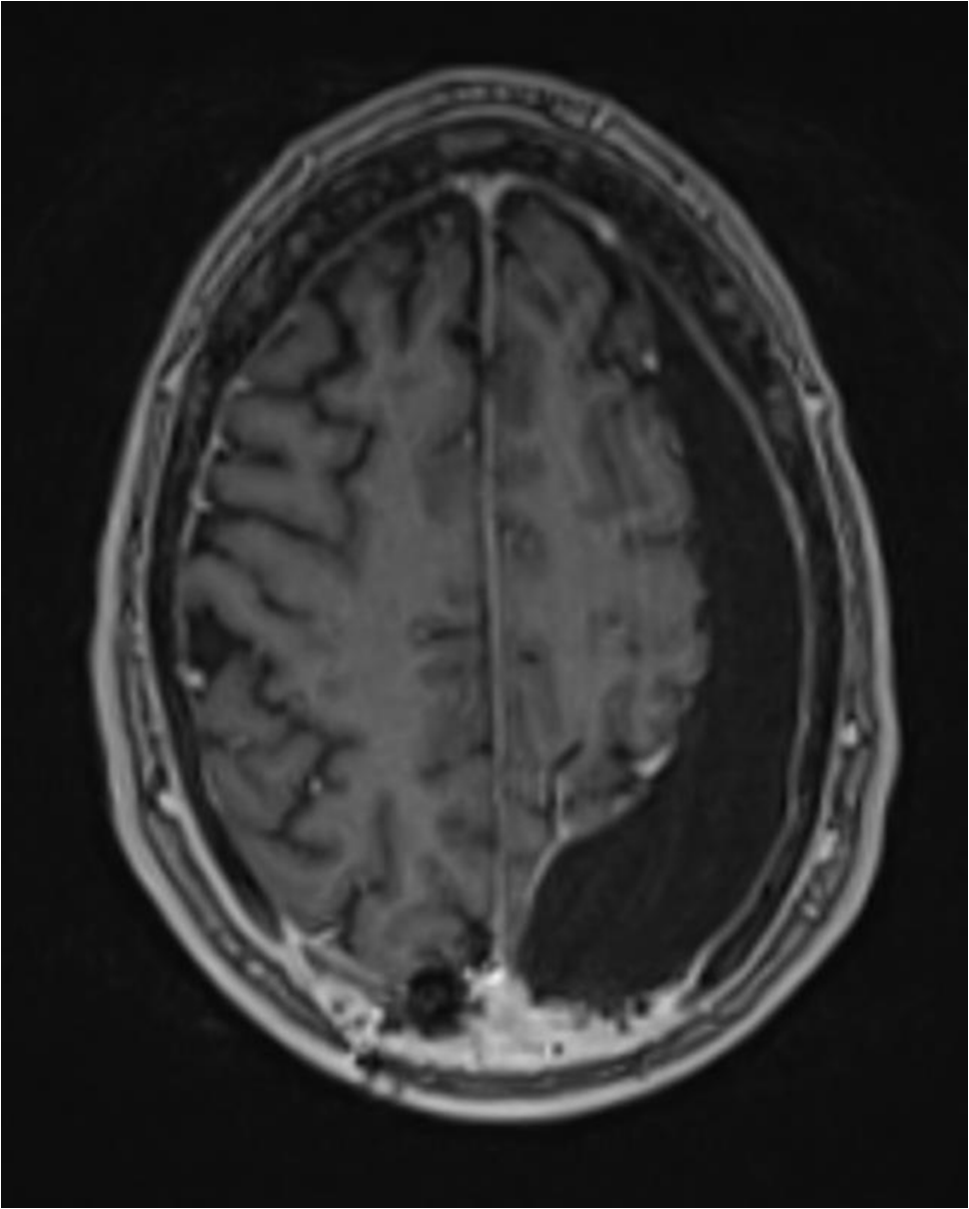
Postoperative axial T1-weighted MRI showing no residual subcutaneous CSF
